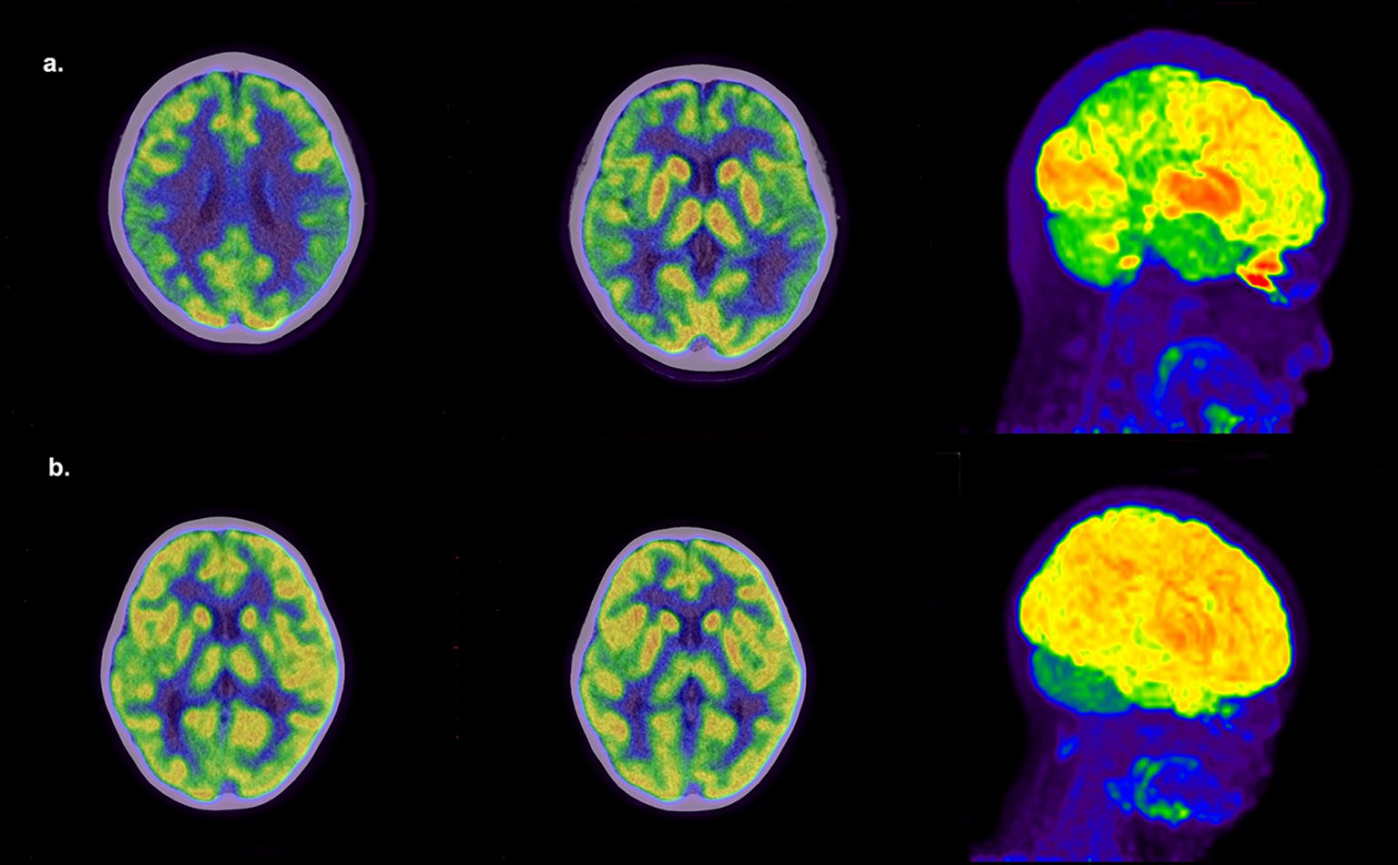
Representative PET images. (a) An 11-year-old girl with AE had a 1-week history of seizures. ^18^F-FDG PET/CT shows extensive hypometabolism in bilateral frontal, parietal, and temporal lobes and hypermetabolism in the basal ganglia and thalamus. (b) A 3-year-old girl with a 2-year history of seizures and developmental delay was finally diagnosed with intractable epilepsy. ^18^F-FDG PET/CT shows hypometabolism in the right temporal lobe and right thalamus.
